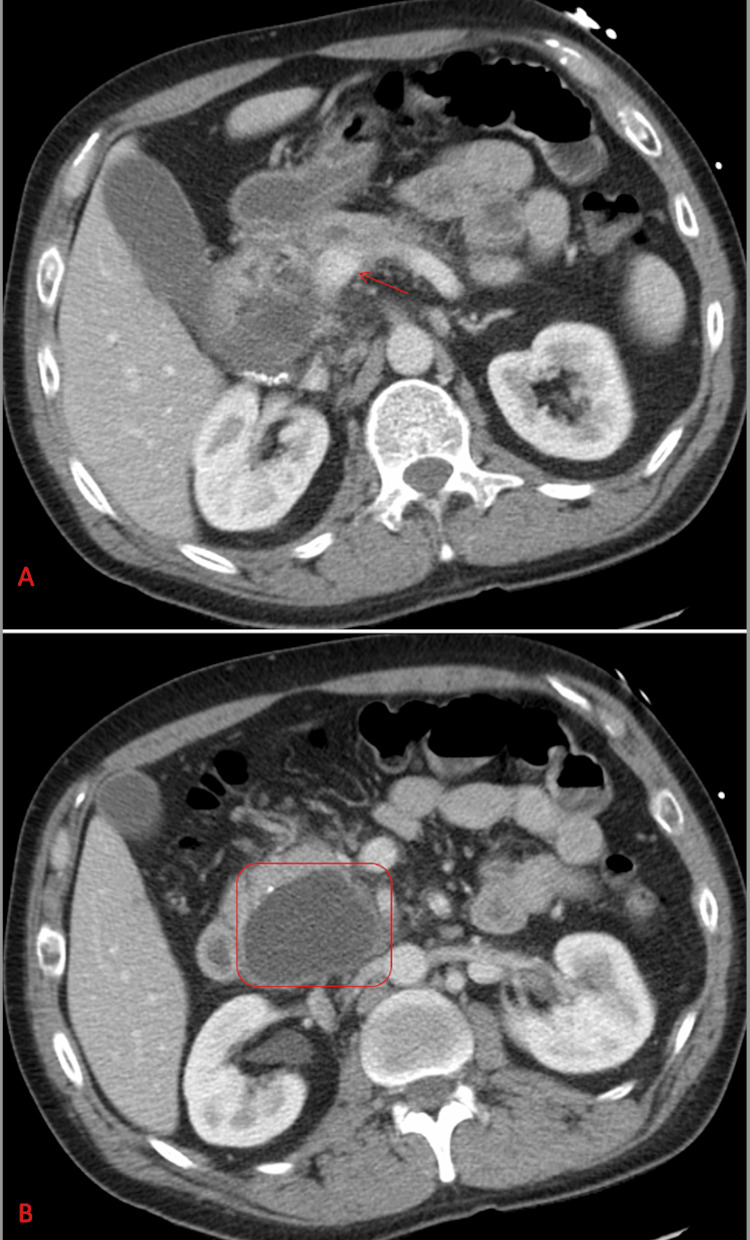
CT abdomen with IV contrast (axial view) showing peripancreatic stranding (red arrow, panel A), pancreatic calcifications, and a pancreatic pseudocyst measuring 6.2 × 4.1 cm (red box, panel B).
